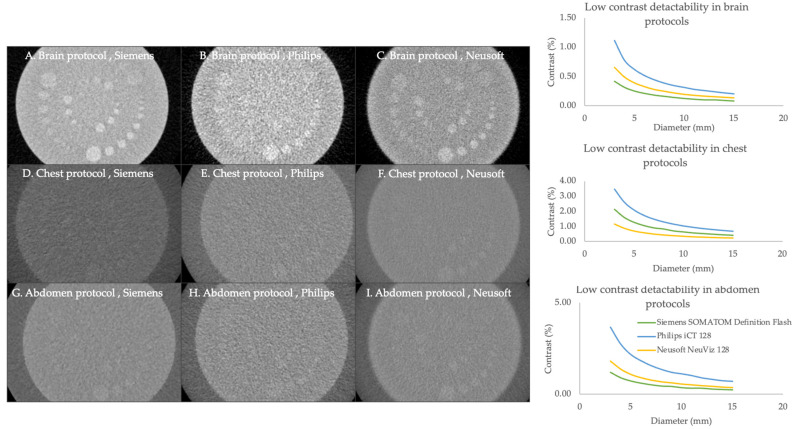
Comparison of low-contrast detectability of brain, chest, and abdomen protocols with various CT scanners. The cylindrical object at various diameter (shown in the white cylindrical dot) is used to analyze low contrast detectability and contrast-to-noise ratio. The green, blue, and yellow lines represent the Siemens SOMATOM Definition Flash, Philips iCT128, and Nuesoft NeuViz 128, respectively.
